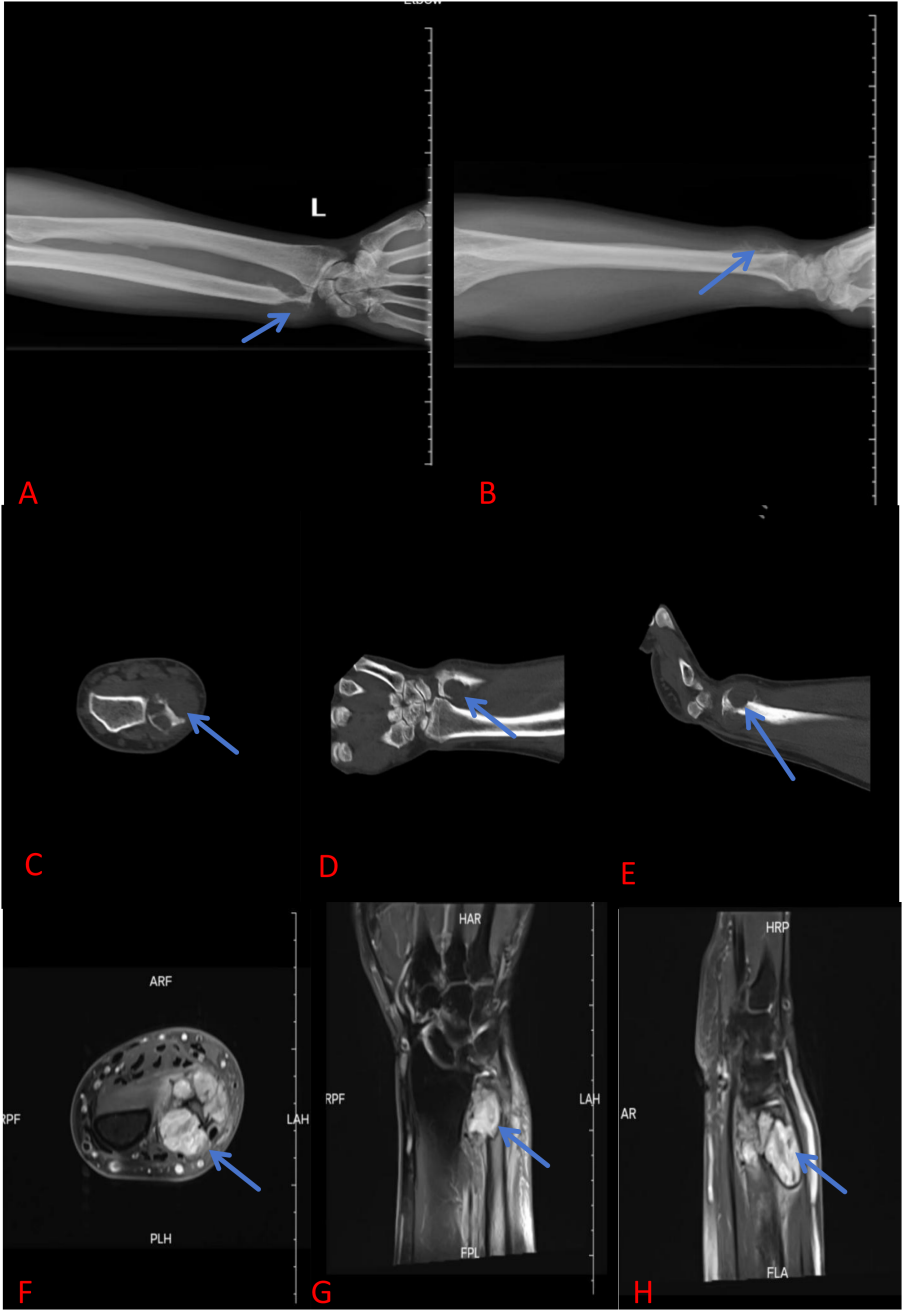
(A) Latera X-ray of the left ulna. (B) Anteroposterior X-ray of the left ulna: Expansive tumor at the distal ulna. Left ulna CT: There is obvious bone destruction at the distal ulna. (C) Transverse position. (D) Coronal position. (E) sagittal position. Left ulna MRI: a soft tissue mass at the distal end of the left ulna, measuring 3.9cm × 3.7cm × 3.5cm. (F) Transverse position. (G) Coronal position. (H) sagittal position.
